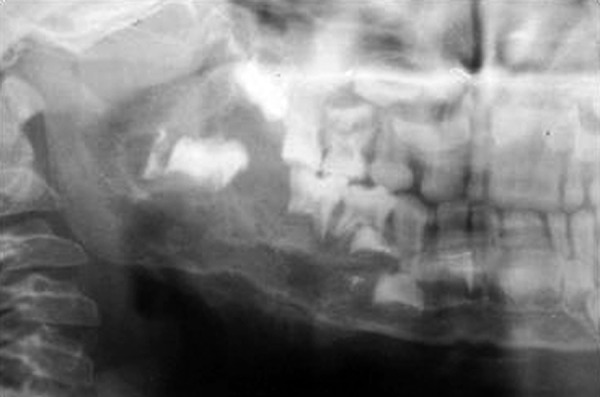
Cropped pretreatment panoramic radiograph shows radiolucent ill-defined lytic lesion with bony spiculations, teeth displacement and missing 45
